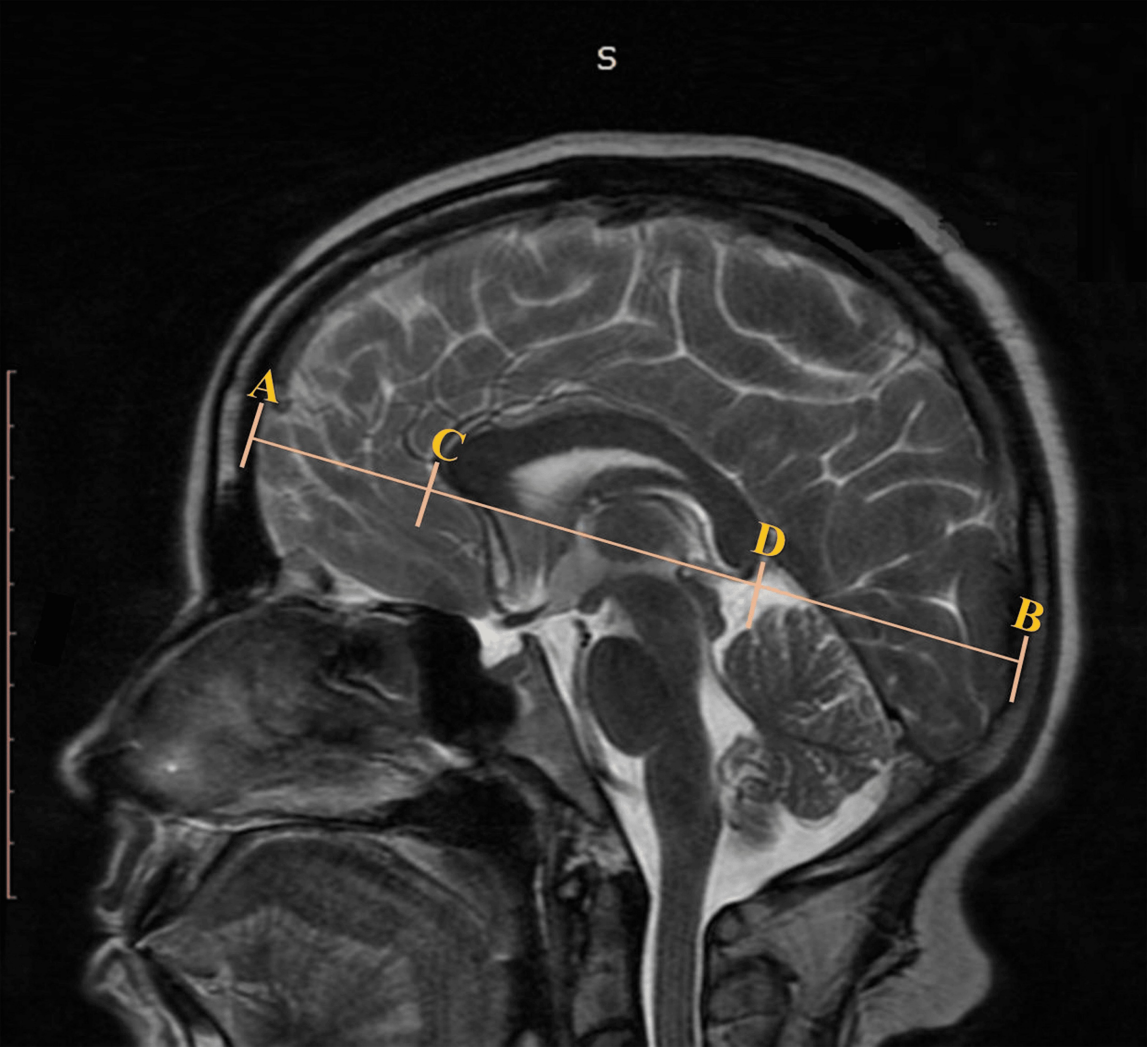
Mid-sagittal T2-weighted MRI of brain shows the main parts of corpus callosum. A- the anterior most point of frontal pole of brain, B- the posterior most point of occipital pole of brain, C - the anterior most part of genu part of corpus callosum, D- the posterior most point of splenium part of corpus callosum, CD- line between the anterior most point of genu (C) to posterior-most point of splenium (D), AB- line between the anterior most point of frontal pole of brain (A) to the posterior most point of an occipital pole of brain (B), AC- line between anterior most point of brain (A) to anterior most point of corpus callosum (C), DB- line between posterior most point of corpus callosum (C) to anterior most point of brain (B), Distance between posterior most point of brain to posterior most point of CC.
